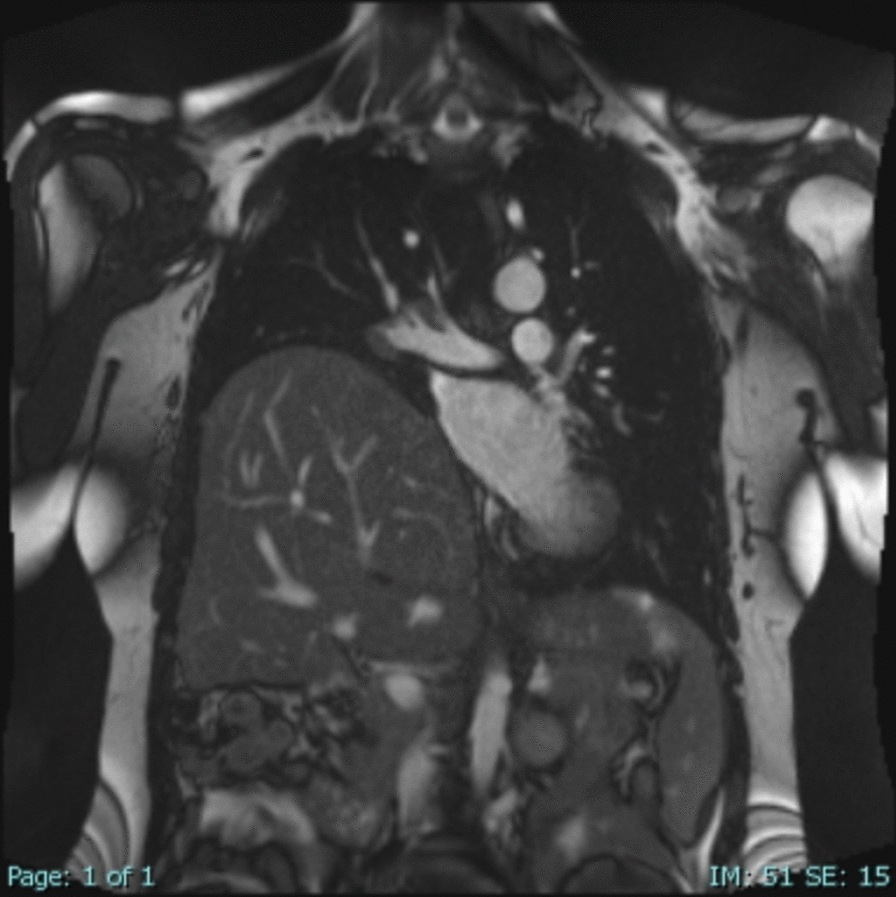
Preoperative dynamic sagittal chest magnetic resonance imaging showing a significantly elevated right hemidiaphragm with reduced mobility
